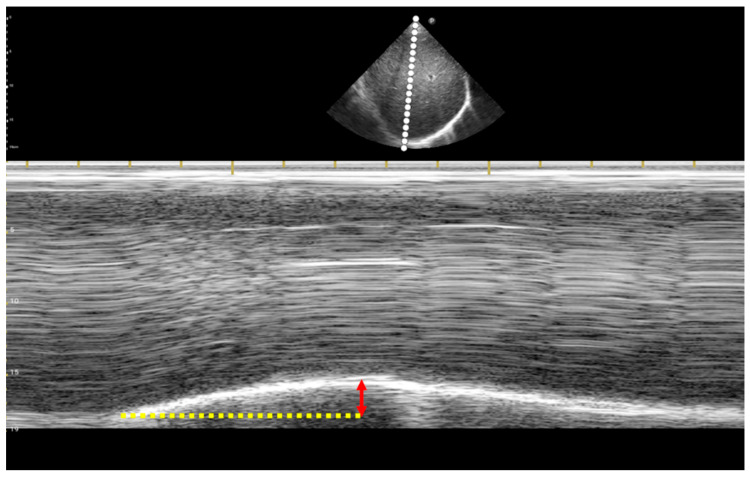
M-mode ultrasound of the diaphragm was used to measure diaphragmatic displacement (DD) and diaphragm inspiratory time (Ti). DD, which corresponds to the vertical distance from the end of the expiratory baseline to the peak inspiratory amplitude, is indicated by the red double-headed arrow, whereas Ti is represented by the yellow dashed line.
